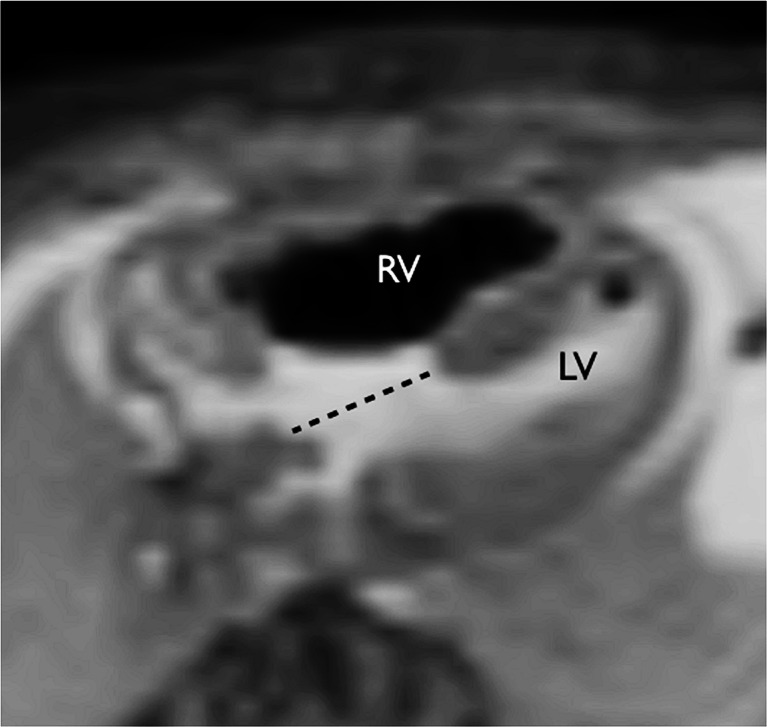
Complete atrioventricular septal defect (*dotted line*) on axial T2-weighted postmortem MRI in a 22-week gestation fetus with trisomy 21. Note air in the right ventricle (*RV*) secondary to fetocide injection and a trace of pericardial effusion. *LV* left ventricle. Reproduced with permission [14]
